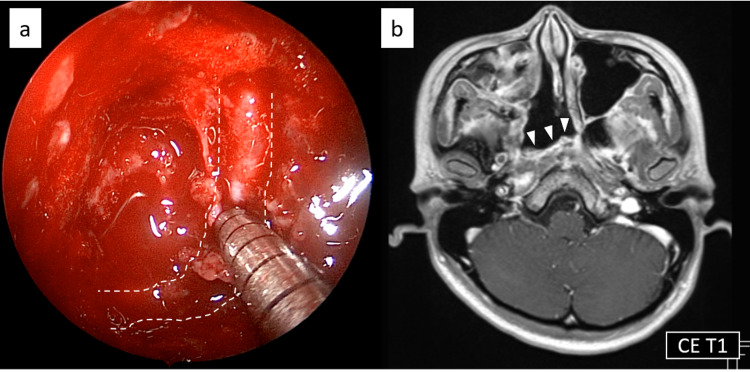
Intraoperative image and postoperative MRI a) Endoscopic image at the end of the surgery. The paraclival portion of the internal carotid artery was exposed. White dotted line shows the course of the internal carotid artery. b) Contrast-enhanced MRI five months after the surgery showed no apparent recurrence. White arrow heads: the pedicled nasal septum flap covering the exposed internal carotid artery.
